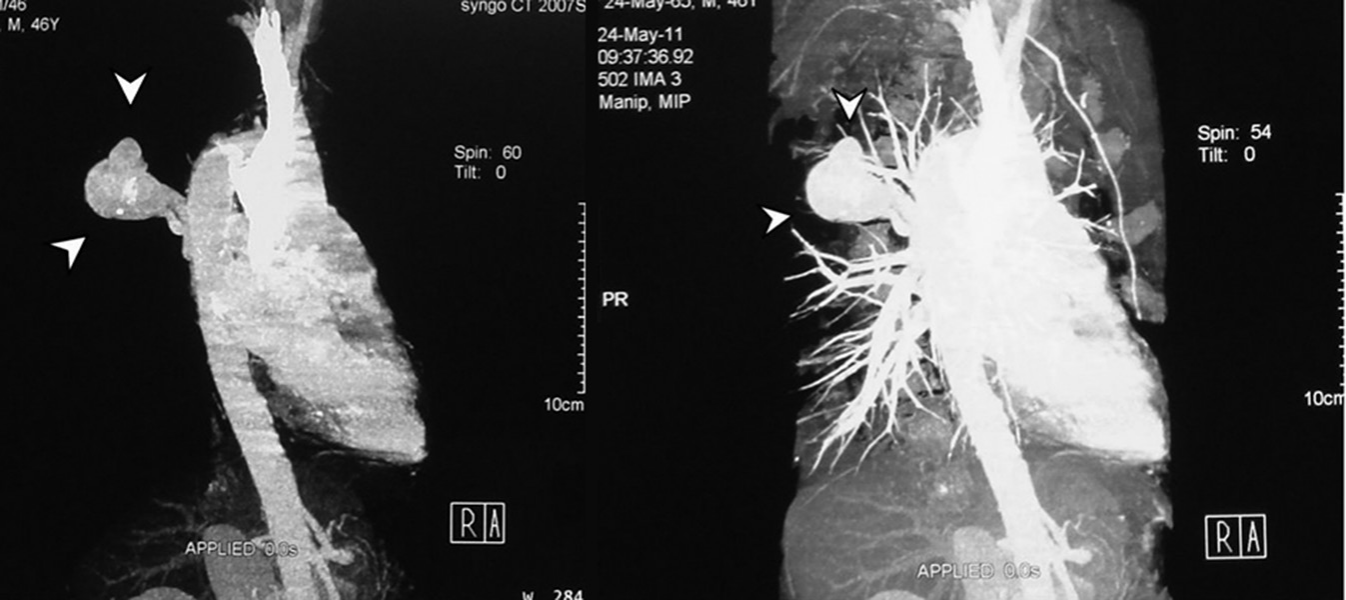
CT angiography after 3 months. No growth or thrombosis was detected.
